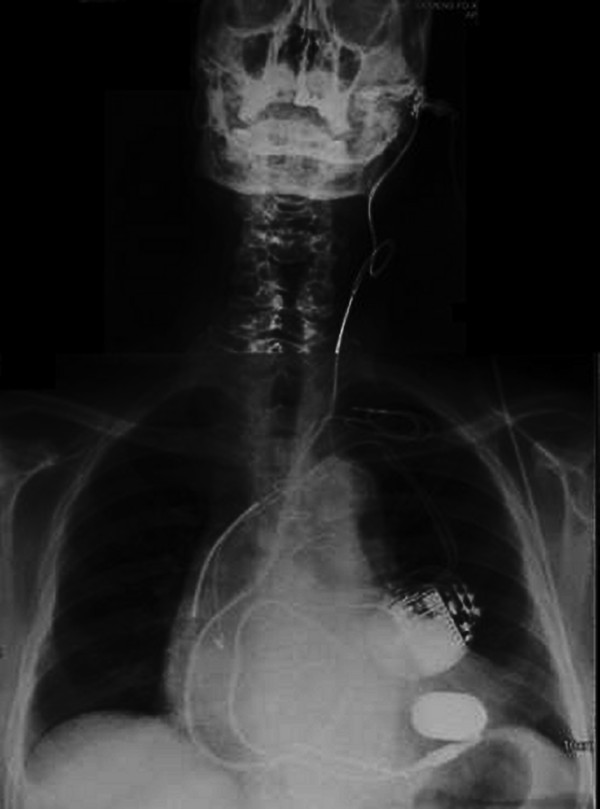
A full range of cardiac support technology. The plain chest x-ray shows a Jarvik pump in the apex of the left ventricle with power cable passing through the neck to the skull pedestal. There is an implantable cardio-defibrillator and dual chamber pacemaker with additional wire for cardiac resynchronisation therapy. There are drug eluting stents in the left coronary artery. Bone marrow stem cells now add a further dimension to supportive therapy.
